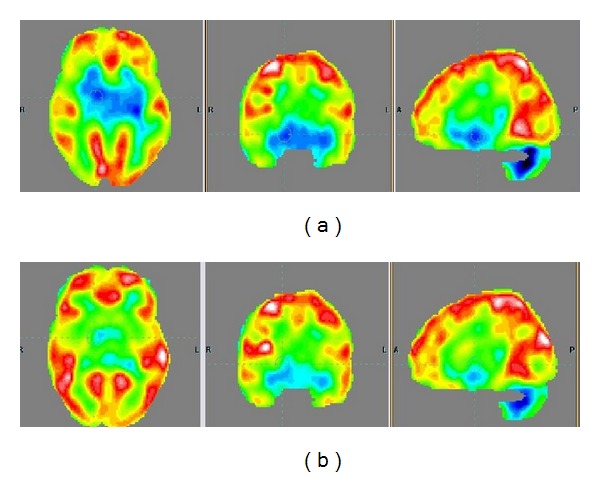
Findings in PET-CT scan before and after cellular therapy. (a) PET-CT scan before intervention showing reduced FDG uptake in the areas of frontal lobe, cerebellum, amygdala, hippocampus, parahippocampus, and mesial temporal lobe. (b) PET-CT scan six months after intervention comparison shows increased FDG uptake in the areas of frontal lobe, cerebellum, amygdala, hippocampus, parahippocampus, and mesial temporal lobe.
